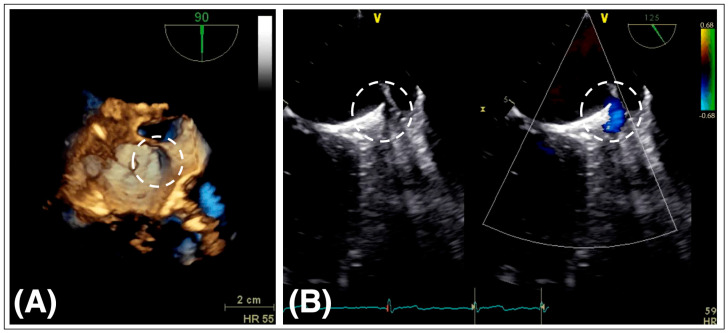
Peri-device leaks. (A) 3D TEE of the left atrium after LAAO with AMPLATZER™ Cardiac Plug and residual PDL (dashed circle), (B) left: 2D TEE after LAAO with incomplete sealing and resulting PDL (dashed circle), right: residual blood flow around the LAAO device edge into the left atrium as seen by color Doppler (dashed circle), TEE transesophageal echocardiography, LAAO left atrial appendage occlusion, PDL peri-device leak.
